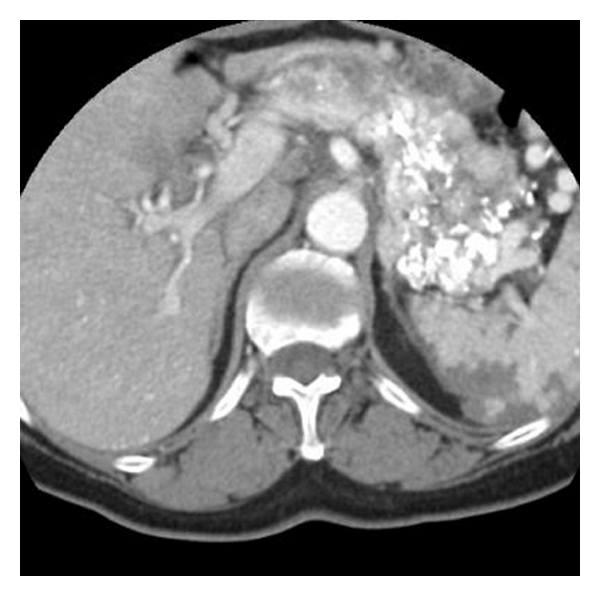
Contrast-enhanced axial CT scan through the pancreas after the embolization of the tumor shows multiple hyperdense embolic particles in the pancreatic tumor and also in the spleen and the right liver lobe.
